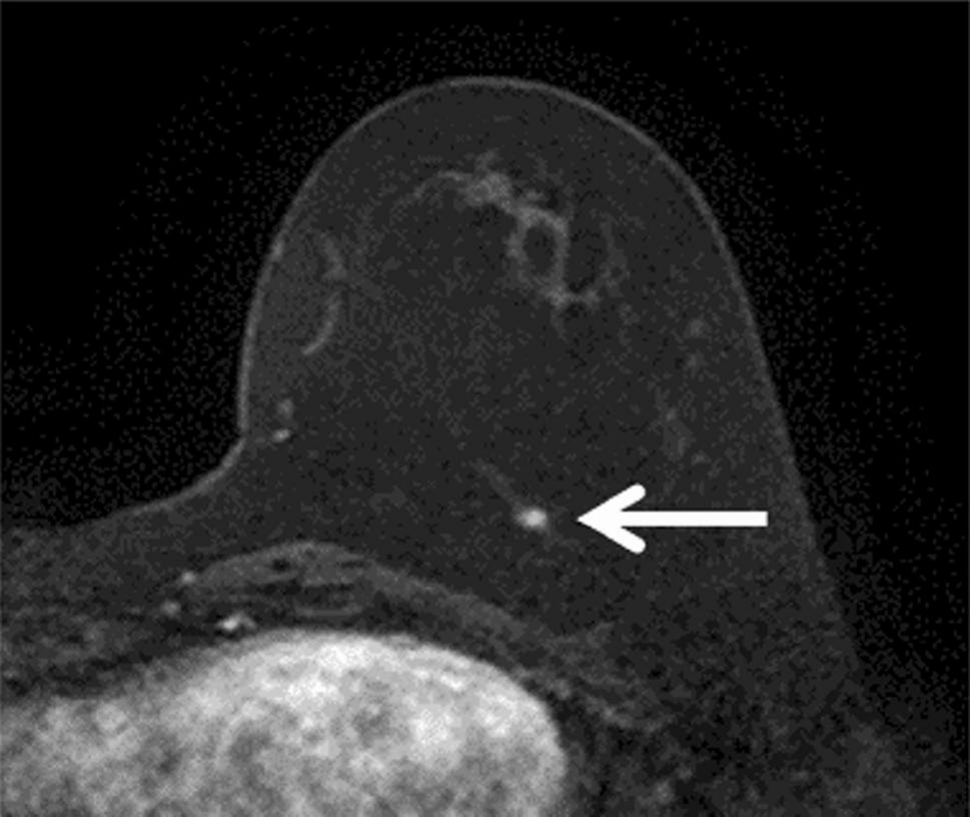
Transverse first dynamic contrast-enhanced MR image in an asymptomatic 52-year-old female BRCA1 mutation carrier for post-operative follow-up evaluation, which revealed a 5 mm mass lesion (arrow) in the left breast. Through MRI-guided biopsy, this case was diagnosed as invasive ductal cancer, triple-negative
